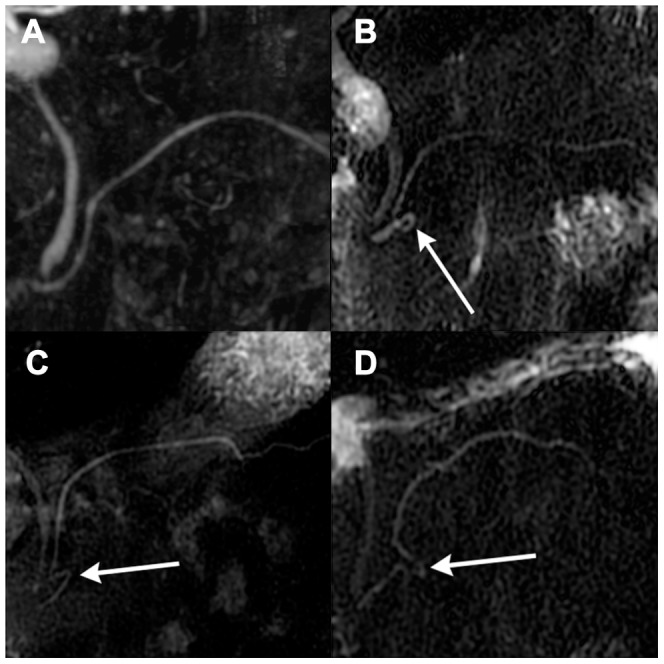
Anatomical variations of meandering main pancreatic duct as seen on magnetic resonance images. (A) Normal type on a magnetic resonance cholangiopancreatography (MRCP) image, (B) loop type on an MRCP image, (C and D) reverse-Z type on MRCP images. The white arrow on subfigures (B, C, D) indicates an abnormally curved section of the main pancreatic duct in the head of pancreas.
